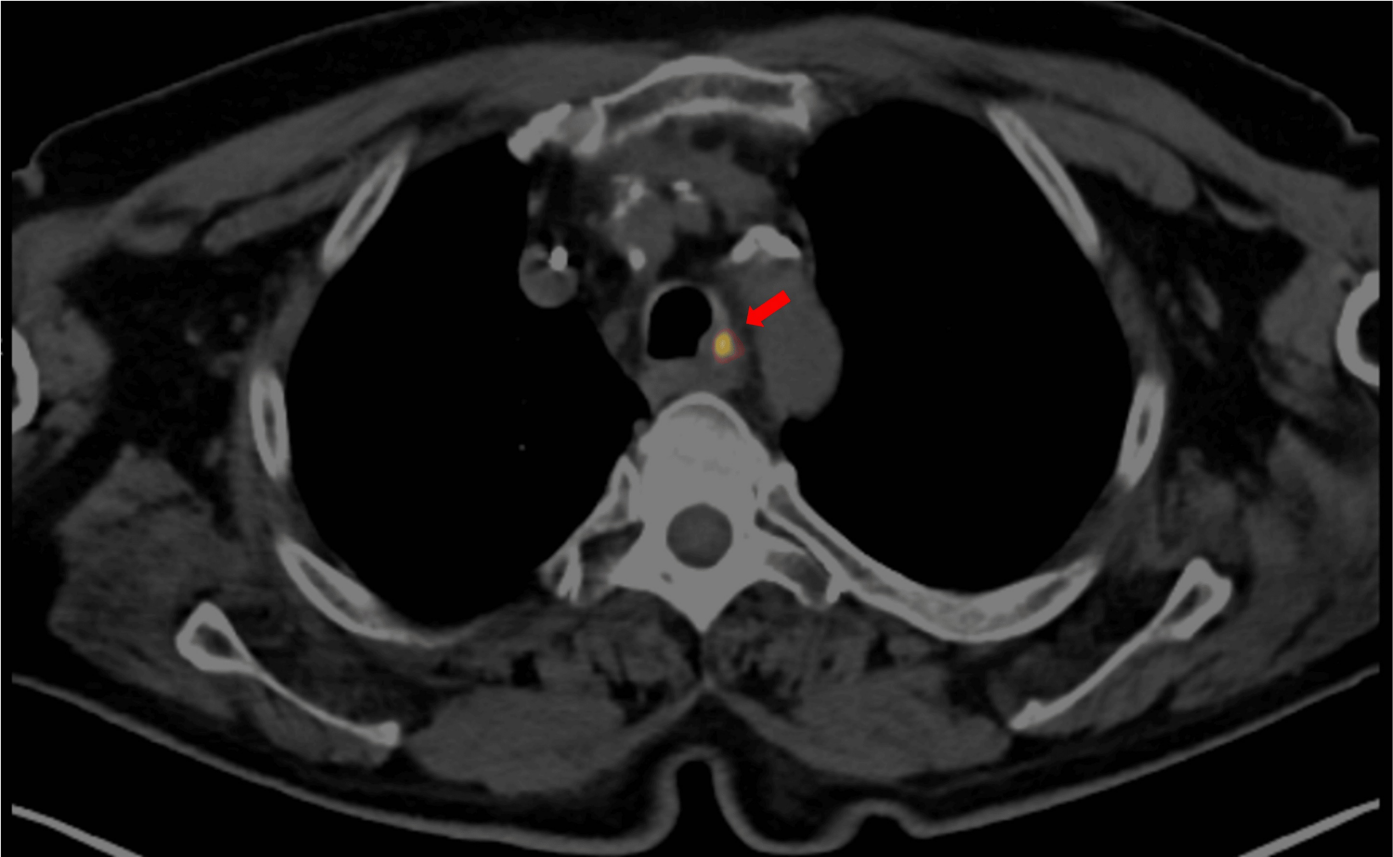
PET-CT scan with a red arrow showing a small focus of FDG uptake in the upper part of the posterior wall of the trachea, along with evidence of endotracheal irregularity PET-CT: Positron Emission Tomography-Computed Tomography; FDG: Fludeoxyglucose
